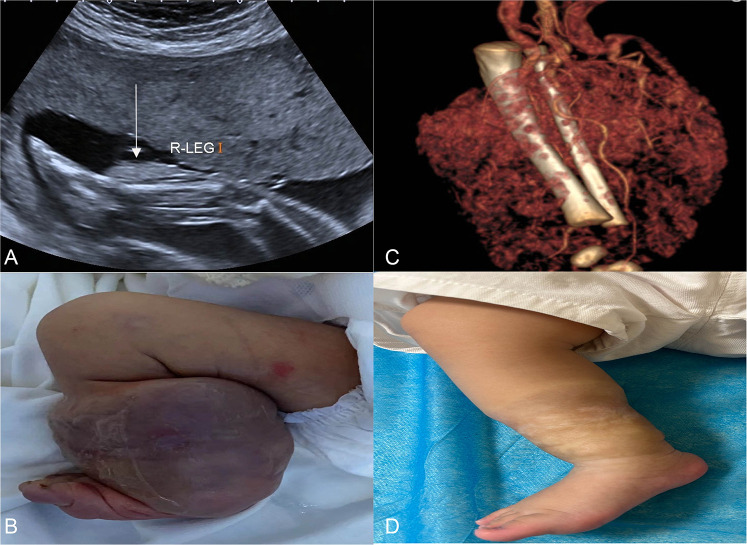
Giant rapidly involuting congenital hemangioma (RICH) of the lower extremity. (A) Prenatal ultrasound reveals a mass with a scalloped appearance, exhibiting echogenicity similar to that of the placenta. (B) A massive dusky purple mass is observed on the right lower leg, with a circumference of approximately 280 mm, surrounded by a peripheral rim of pallor. (C) Contrast-enhanced CT scan reveals tortuous feeding arteries and draining veins within the mass, demonstrating a rapid arterial enhancement with subsequent washout pattern, without involvement of the muscle or bone. (D) The mass showed spontaneous regression at the one-year follow-up.
